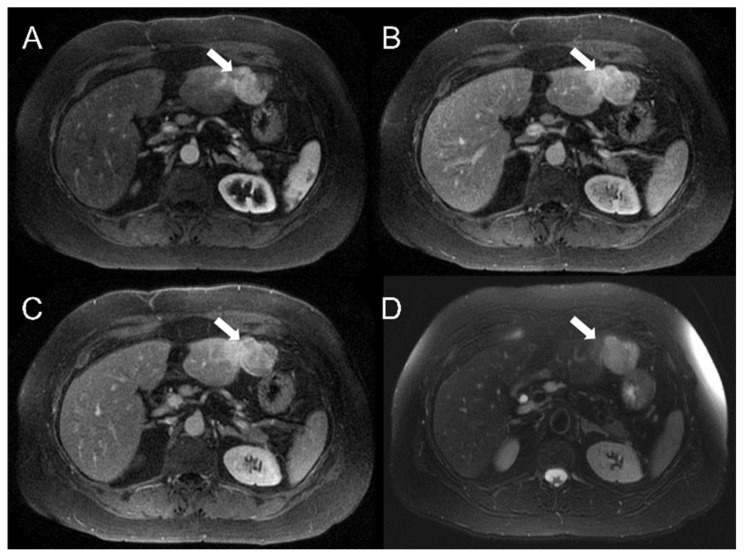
Poorly differentiated HCC (G3) in the left hepatic lobe of a 57-year-old white female. Results showed hyperintense HCC on axial dynamic acquisition (A–C) and on fat-suppressed axial T2-weighted sequence (D) (white arrow).
